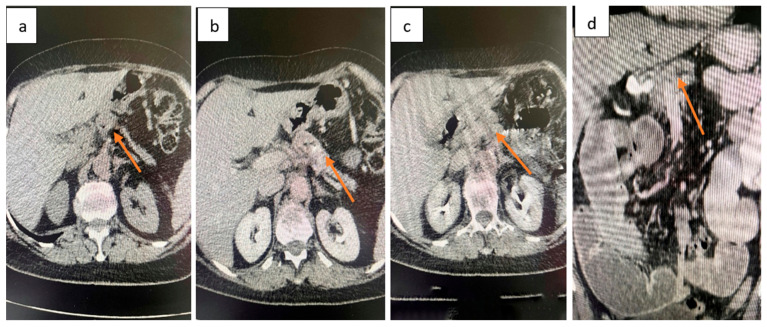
Contrast-enhanced abdominal CT. At the pancreatic body, a spontaneously isodense lesion with discrete iodophilia was identified, measuring 3.2 cm in axial diameter, without ductal dilatation (orange arrow). (a) Non-contrast phase. (b) Arterial phase. (c) Venous phase. (d) Delayed phase.
